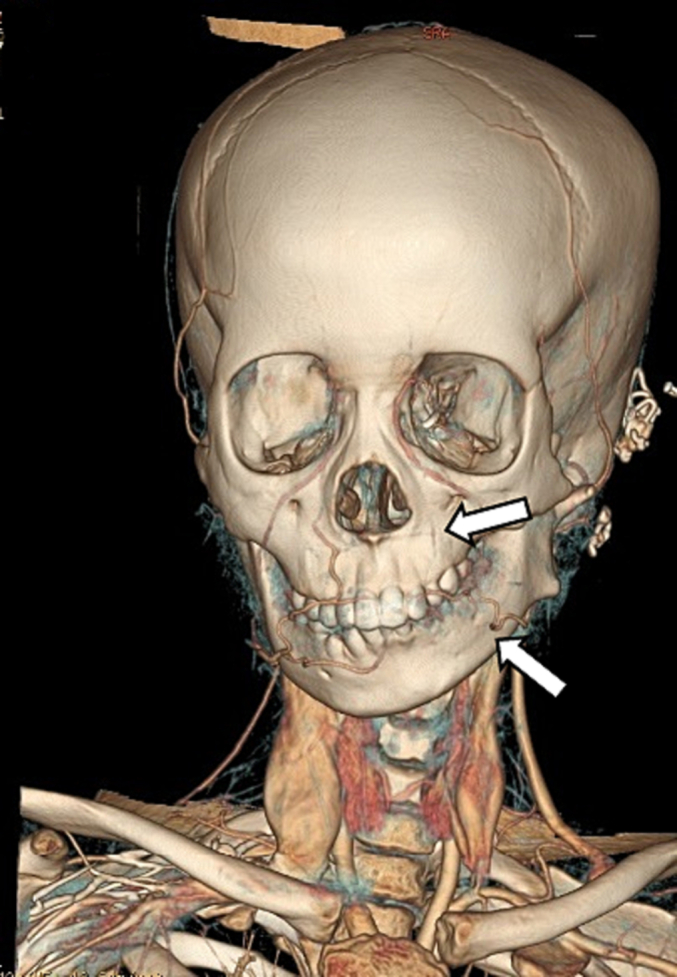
From the CT-Angiography there is agenesis of left submental artery and hypoplasia of left angularis artery.
